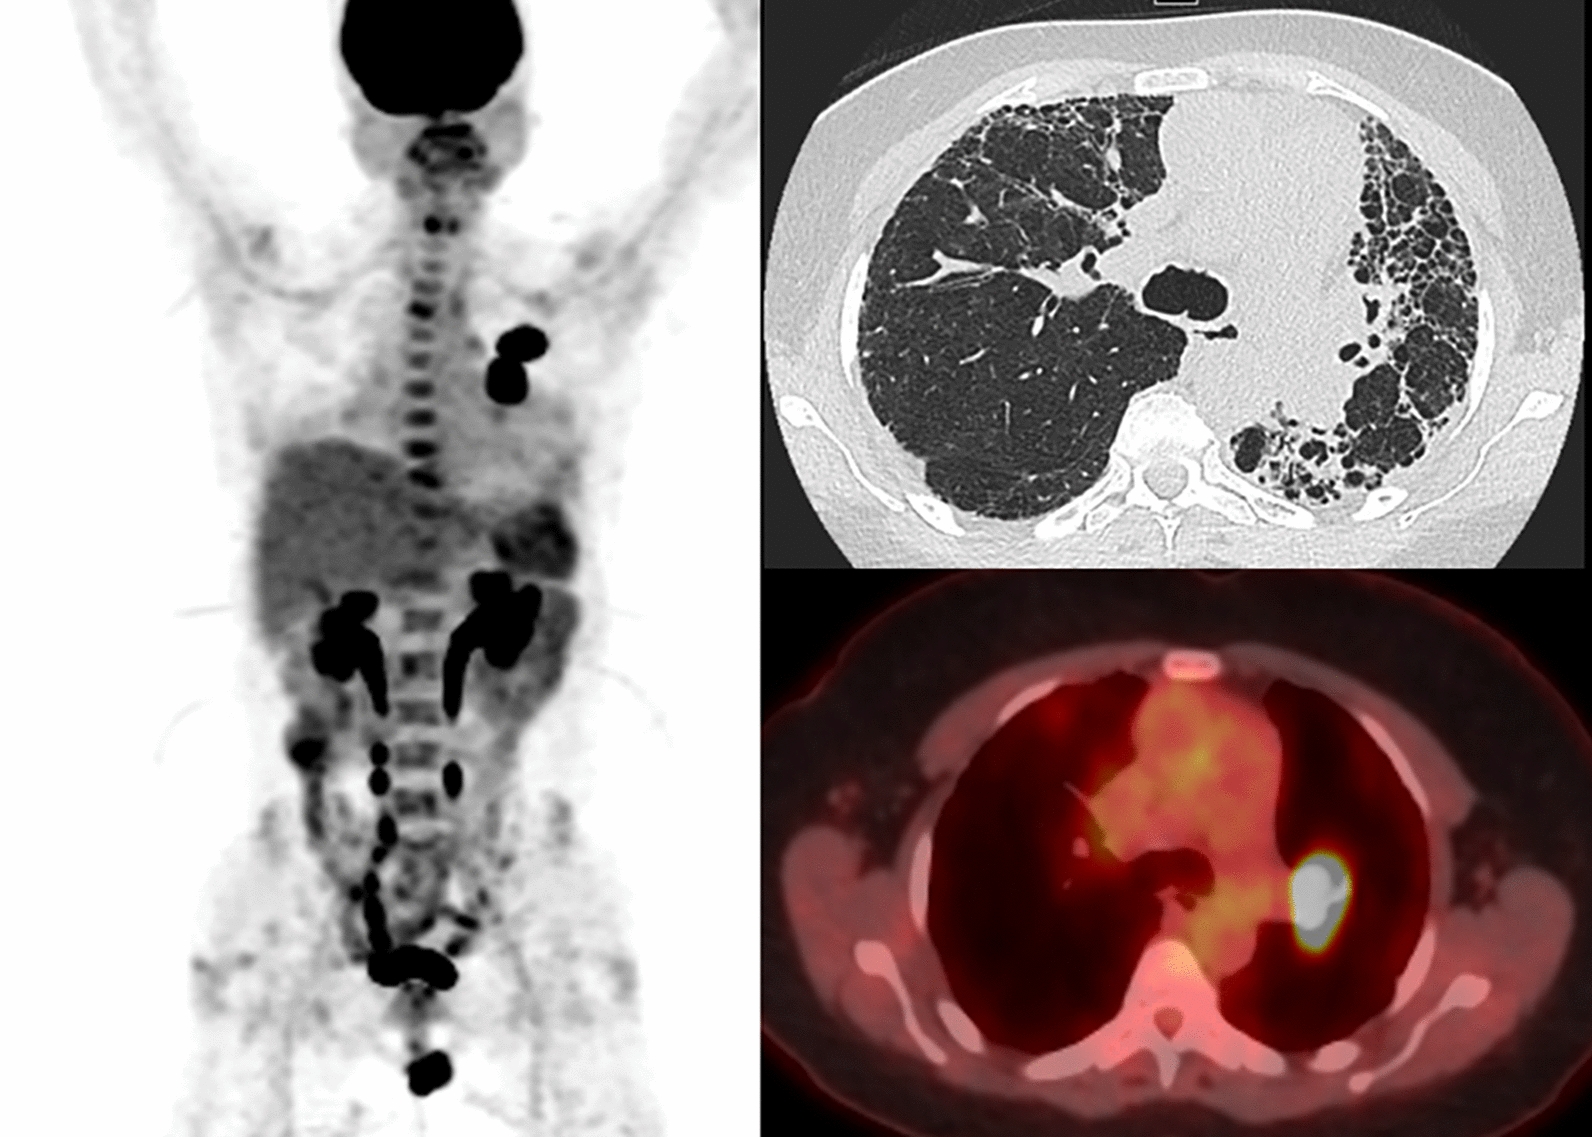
69-year-old man with worsening dyspnea. Maximum intensity projection (MIP), axial CT image and fused axial FDG PET/CT demonstrating heterogeneous FDG uptake corresponding to reticular and linear opacities and areas of honeycombing corresponding to pulmonary fibrosis with focal intense left perihilar FDG uptake corresponding to mass on CT, subsequently biopsied and consistent with adenocarcinoma. Multiple additional FDG avid vertebral lesions were consistent with metastases and CT occult on prior conventional imaging. Low level FDG uptake in the periphery correlates with areas of fibrosis on CT
